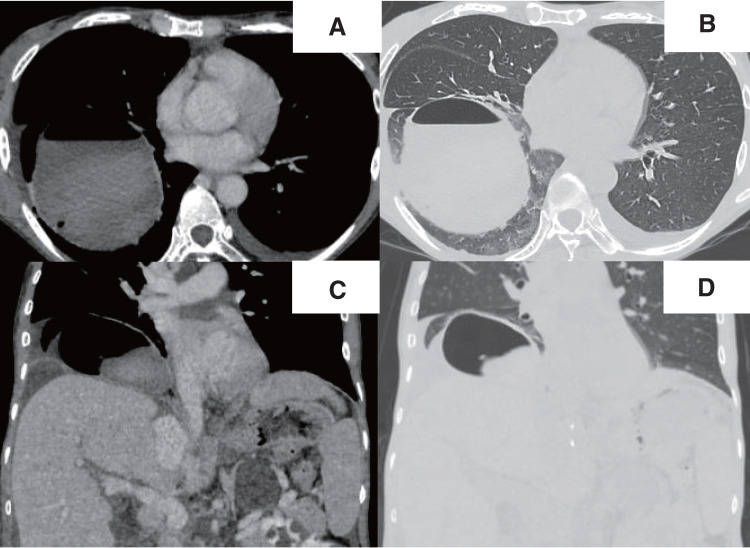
Contrast-enhanced CT images taken just before thoracoscopic surgery, and a large cystic lesion with an air–fluid level was observed adjacent to the right diaphragm. Axial view using a soft tissue window setting (A) and lung window setting (B), and coronal view using a soft tissue window setting (C) and lung window setting (D). CT, computed tomography
